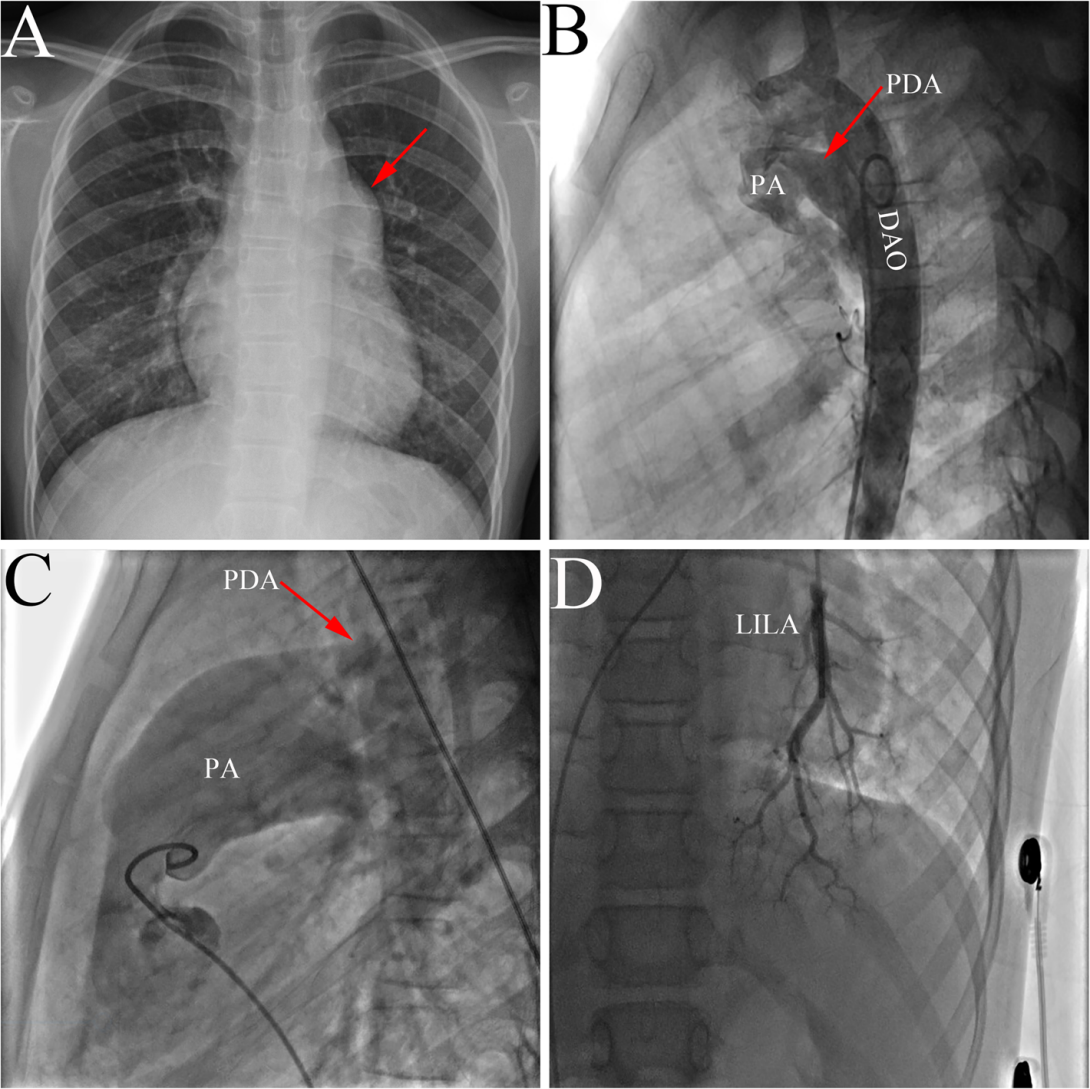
The representative image of PAH-PDA. a: Chest X-ray showed a bulging pulmonary artery at the upper left cardiac border; b: Descending aortography revealed a large PDA; c and d: Pulmonary arteriography showed a large PDA and decreased pulmonary capillary filling.. PAH: pulmonary arterial hypertension; PDA: patent ductus arteriosus; PA: pulmonary artery; DAO: descending aorta; LILA: left inferior lobe artery
